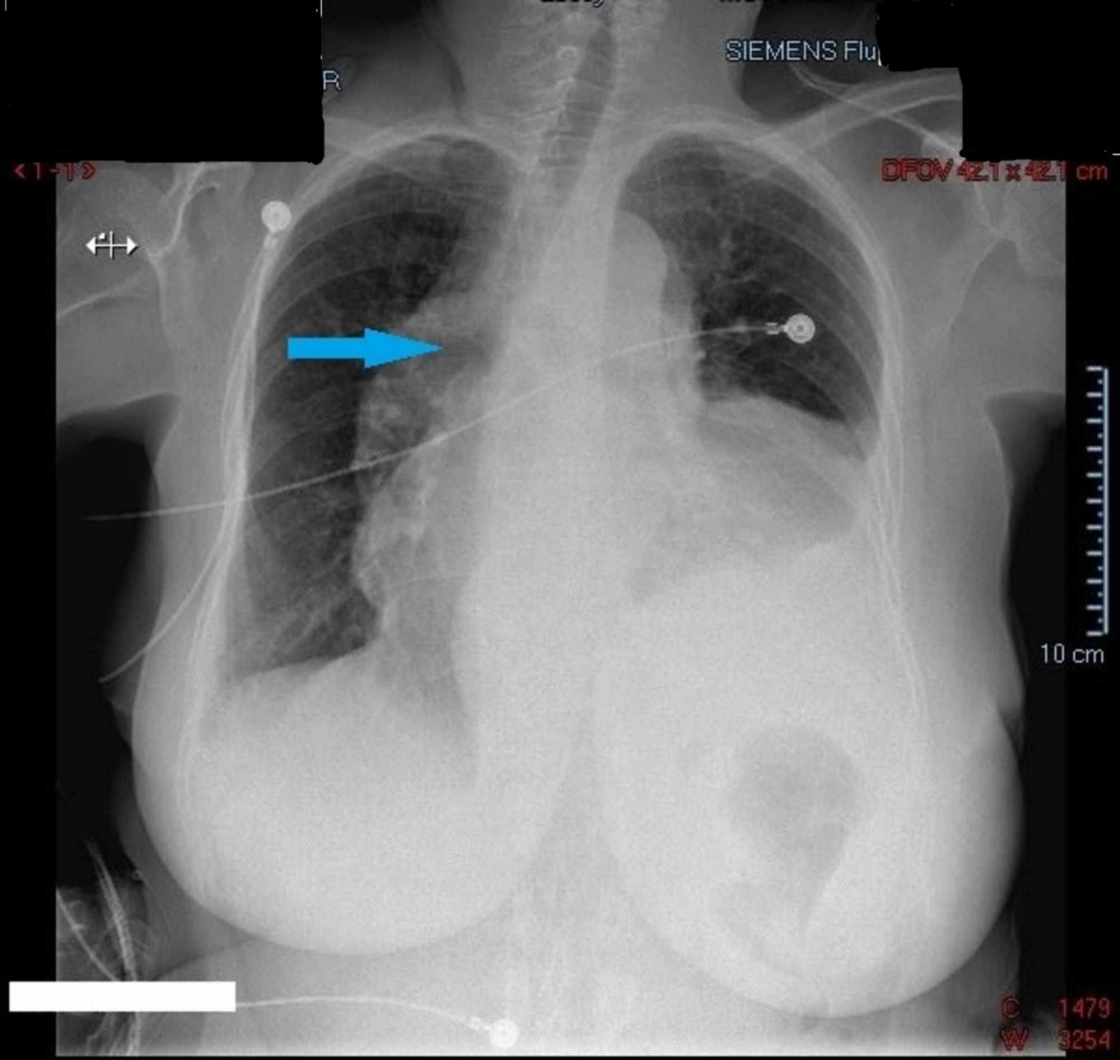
Chest x-ray shows widened mediastinum (blue arrow).
